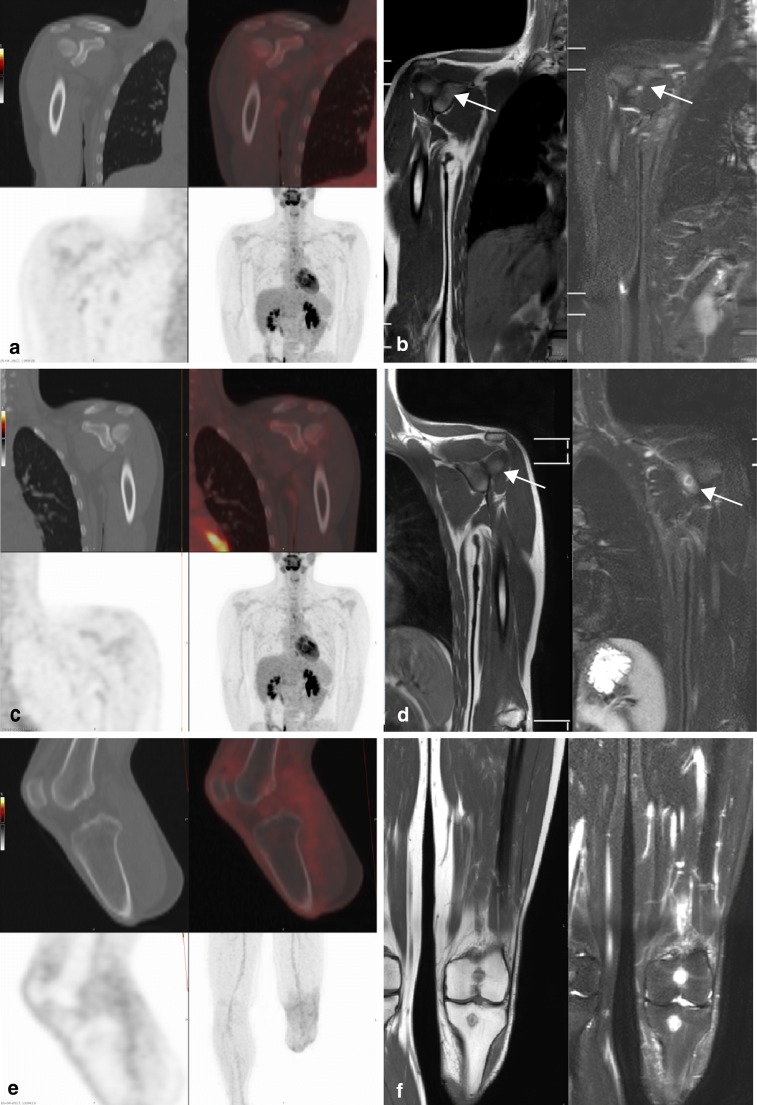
False-negative lesions on ^18^F-FDG PET-CT. A 17-year-old boy diagnosed with localized Ewing sarcoma of the distal tibia. Images obtained 1 year after finishing treatment (6 × VIDE, amputation, 8 × VAI). a^18^F-FDG PET-CT showing no increased ^18^F-FDG-uptake at the glenoid of the right shoulder. b T1-weighted (*left*) and STIR (*right*) images showing a small nodule (*arrow*) with a high degree of suspicion for metastasis at the glenoid of the right shoulder. c^18^F-FDG PET-CT showing no increased ^18^F-FDG-uptake or lytic changes on low-dose CT at the glenoid of the left shoulder. d T1-weighted (*left*) and STIR (*right*) images showing a nodule (*arrow*) with a high degree of suspicion for metastasis at the glenoid of the left shoulder. e^18^F-FDG PET-CT showing no increased ^18^F-FDG-uptake or lytic changes on low-dose CT at the left proximal tibia and distal femur. f T1-weighted (*left*) and STIR (*right*) images showing two nodules with a high degree of suspicion for metastasis at the left proximal tibia and distal femur
